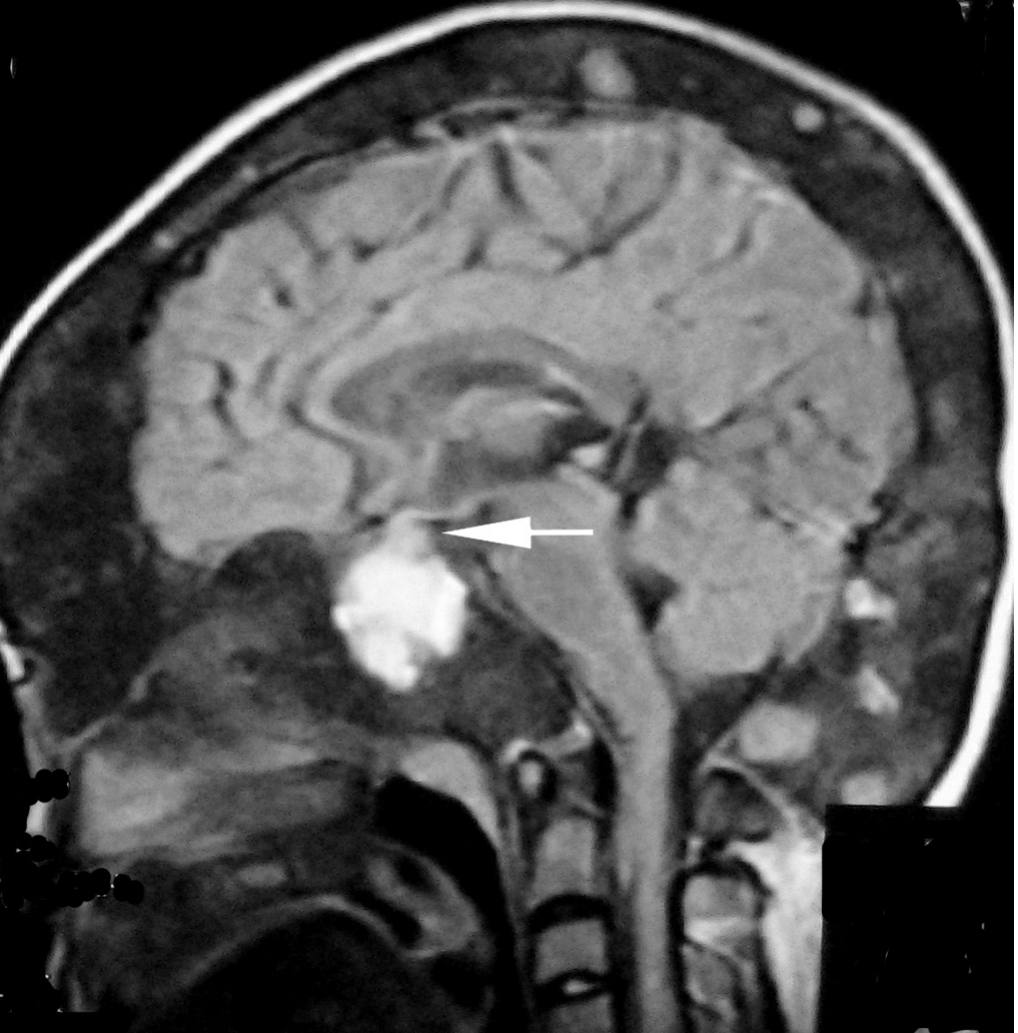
T1-weighted coronal MRI scans of the head. Normal pituitary gland can be found (arrow). The fluid-filled nature of the lesion is demonstrated by the fluid-fluid level.
